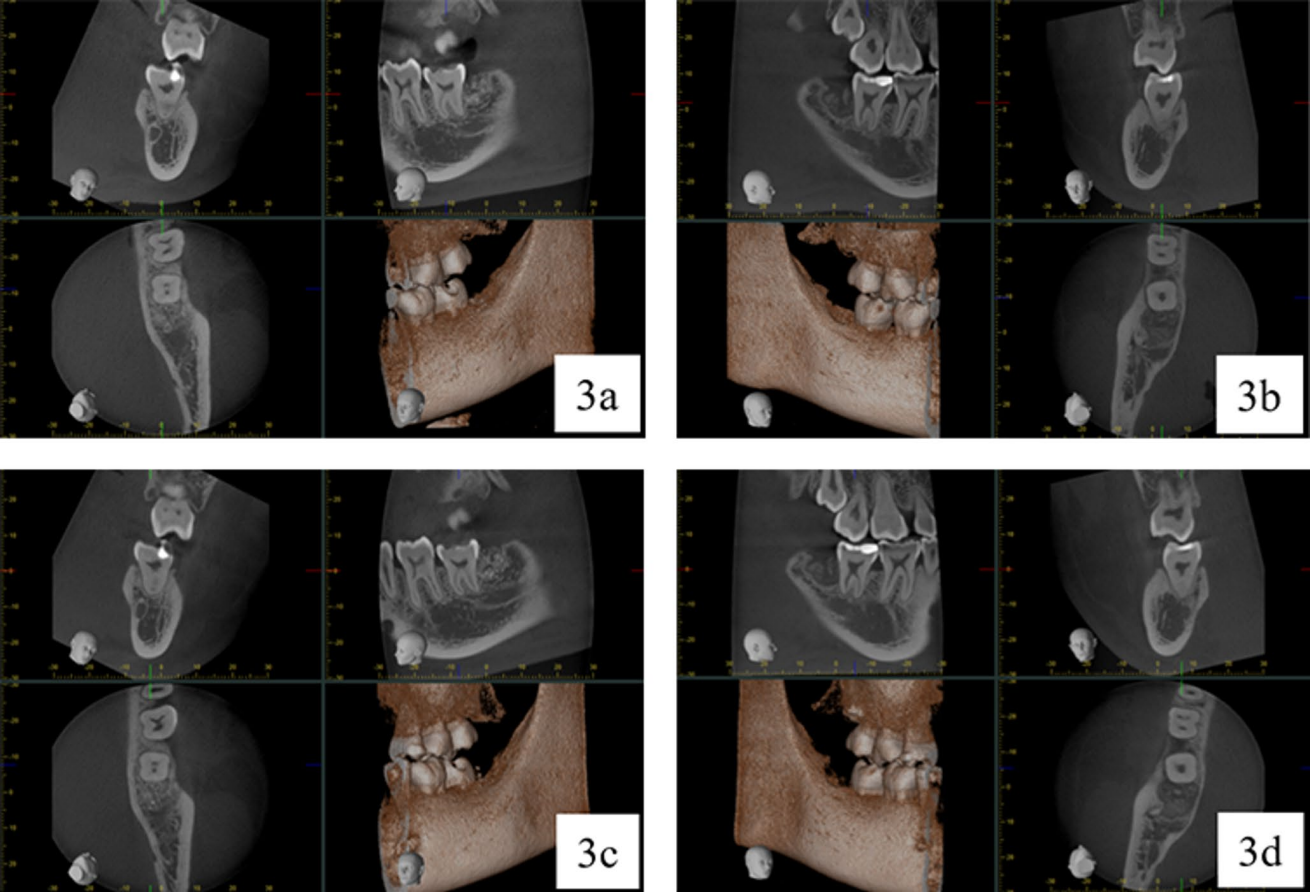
CBCT analysis revealed partial osseointegration of ATSG at 3 months post-operatively (42.86%); (3a), while the xenogenic graft demonstrates no osseointegration during the same period (28.57%); (3b). At the 6-month follow-up, complete osseointegration of the ATSG is observed (64.29%); (3c), whereas the xenogenic graft continues to show no evidence of osseointegration (14.29%);(3d)
